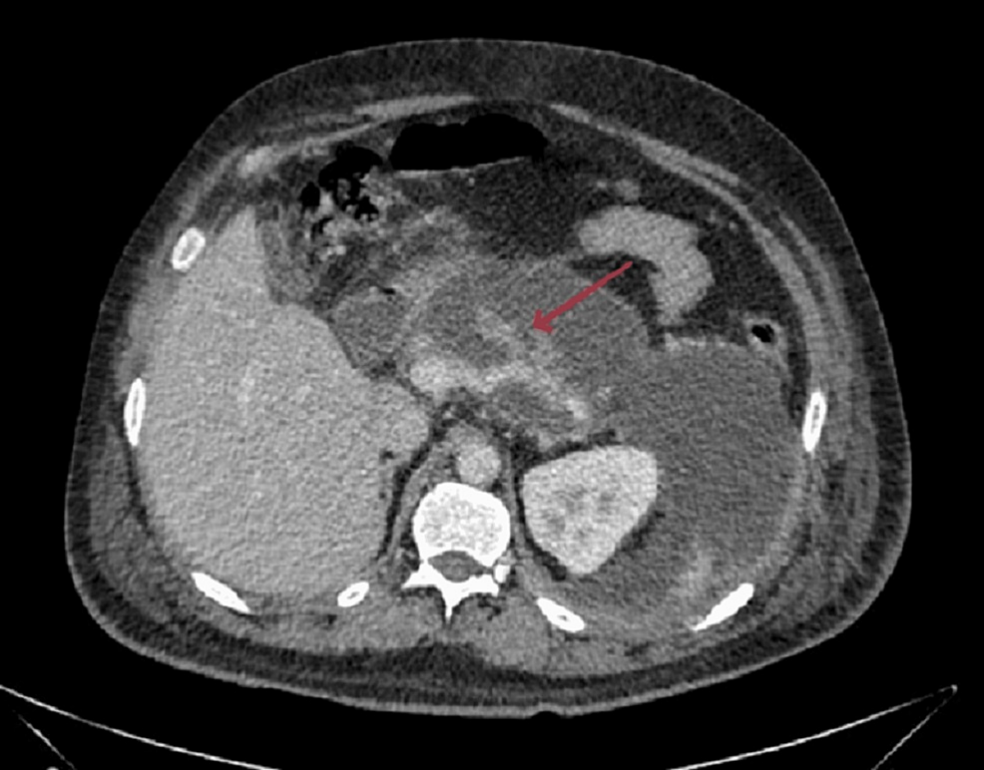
Axial contrast-enhanced CT showing multiple hypodense areas in the body region of pancreas (red arrow) - changes of necrotizing pancreatitis. Ill-defined diffuse fluid collection with enhancing wall in the anterior peripancreatic region extending along the greater curvature of the stomach, peripancreatic region.
